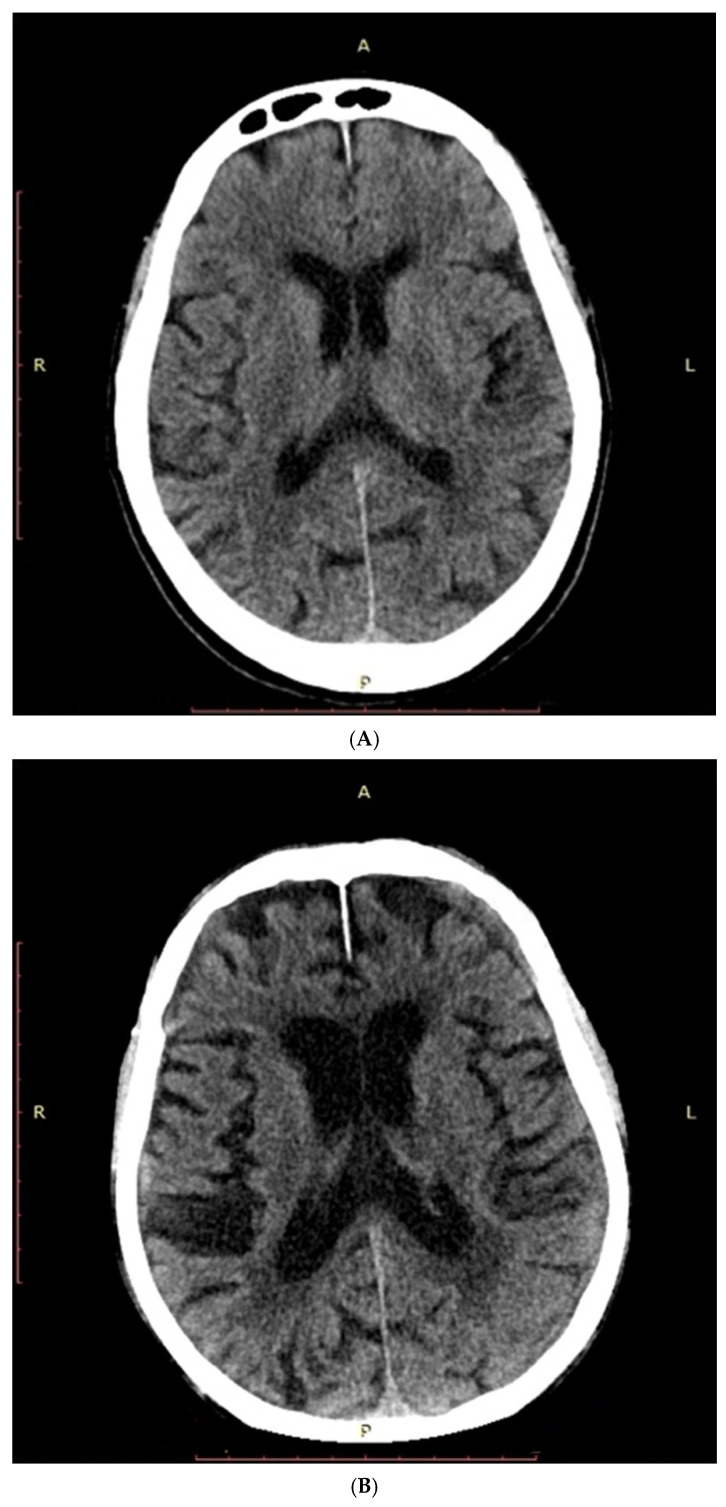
(A) Axial section of the brain on non-contrast CT (radiological image of a normal distribution of brain tissue). (B) Axial section of the brain on non-contrast CT (radiological image of cortical–subcortical atrophy with enlargement of intracranial fluid spaces, typical of brain atrophy). Own material. A—Anterior; L—Left; P—Posterior; R—Right.
